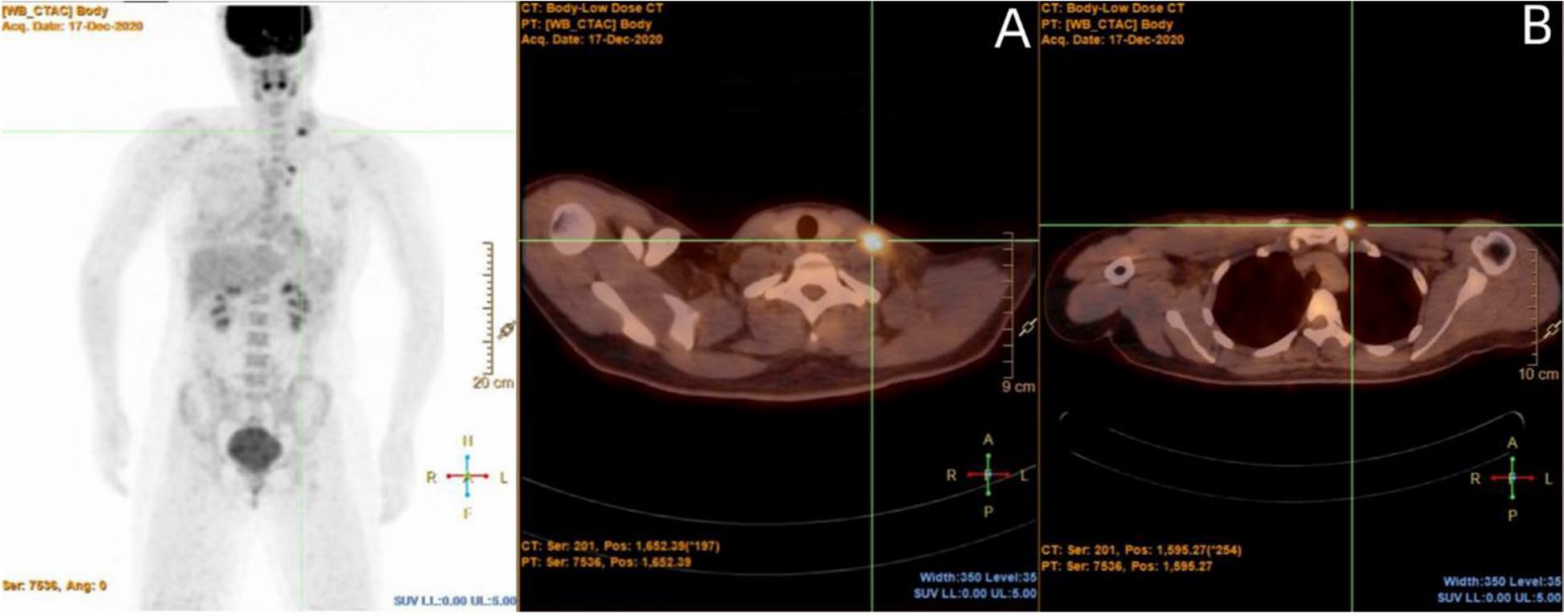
Whole-body PET-CT: There were high metabolic lymph nodes in the left neck and high metabolic nodules on the left side of the sternum stem
